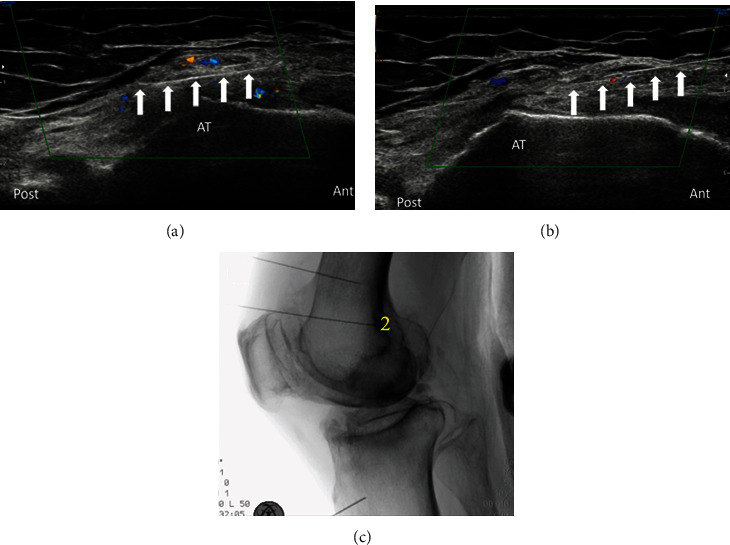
(a) Denervation of the infrapatellar branch of the saphenous nerve (IPBSN) posterior to adductor tubercle. (b) Denervation of superomedial genicular nerve (SMGN) anterior to adductor tubercle. Note the Doppler signals that signify the vessels accompanying the nerves. AT: adductor tubercle; white arrow: Nimbus® radiofrequency needle. (c) A lateral view of fluoroscope of the knee with needle placement for SMGN (more anterior) and IPBSN (more posterior) denervation as shown at 2.
